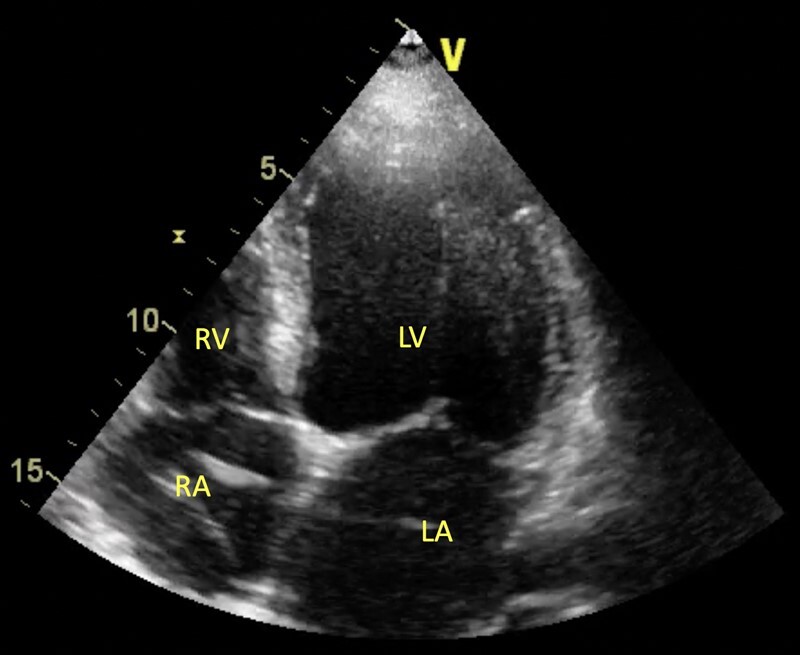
Preliminary scheme. Transthoracic echocardiography, four-chamber view. On admission during the first hospitalization in 2021. No pathological abnormalities visible. LA, left atrium; LV, left ventricle; RA, right atrium; RV, right ventricle.
